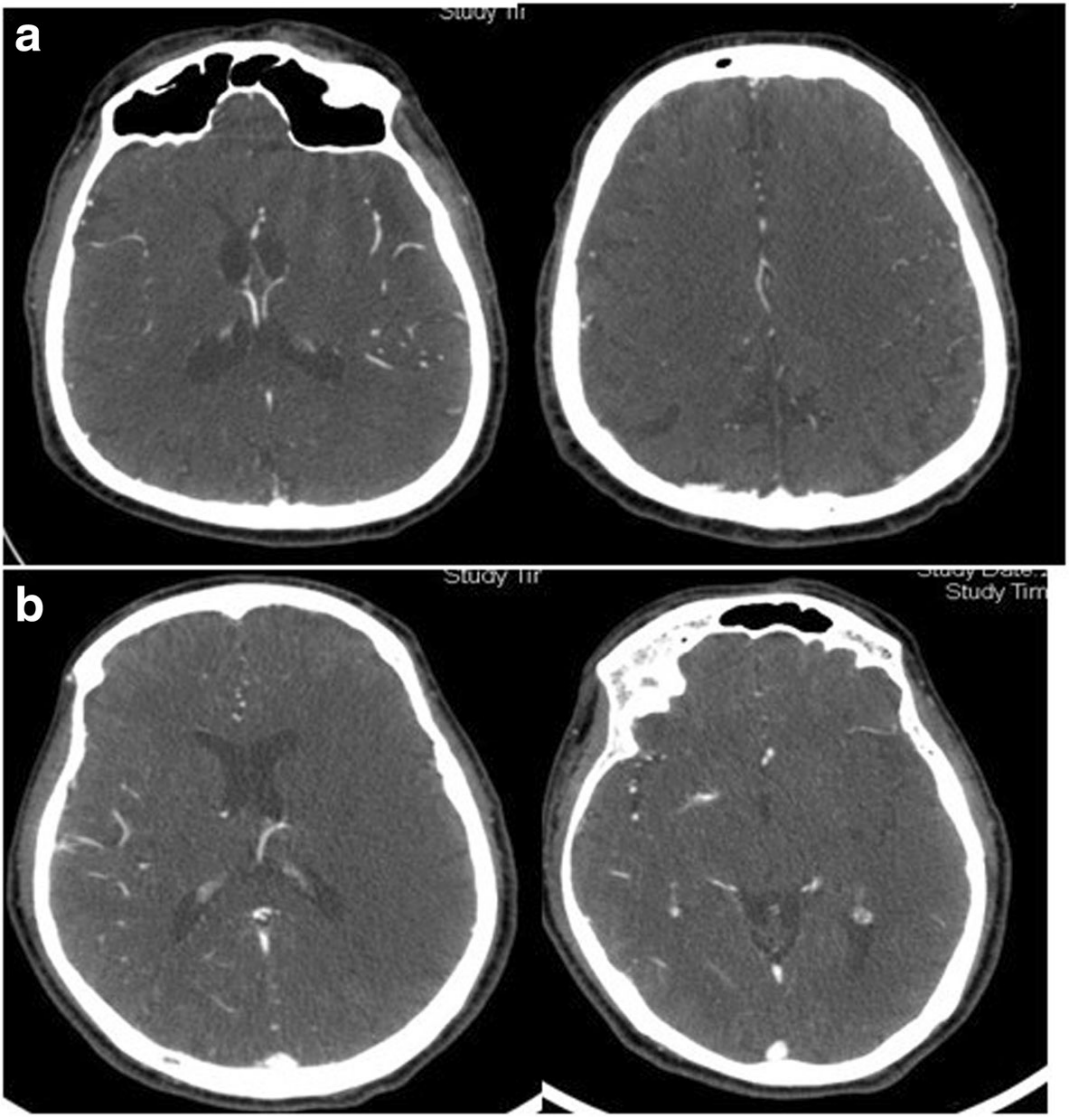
Representative CT images of the collateral circulation grading. a Good collateral grade (6), b poor collateral grade (2). Both patients presented with left MCA occlusion
